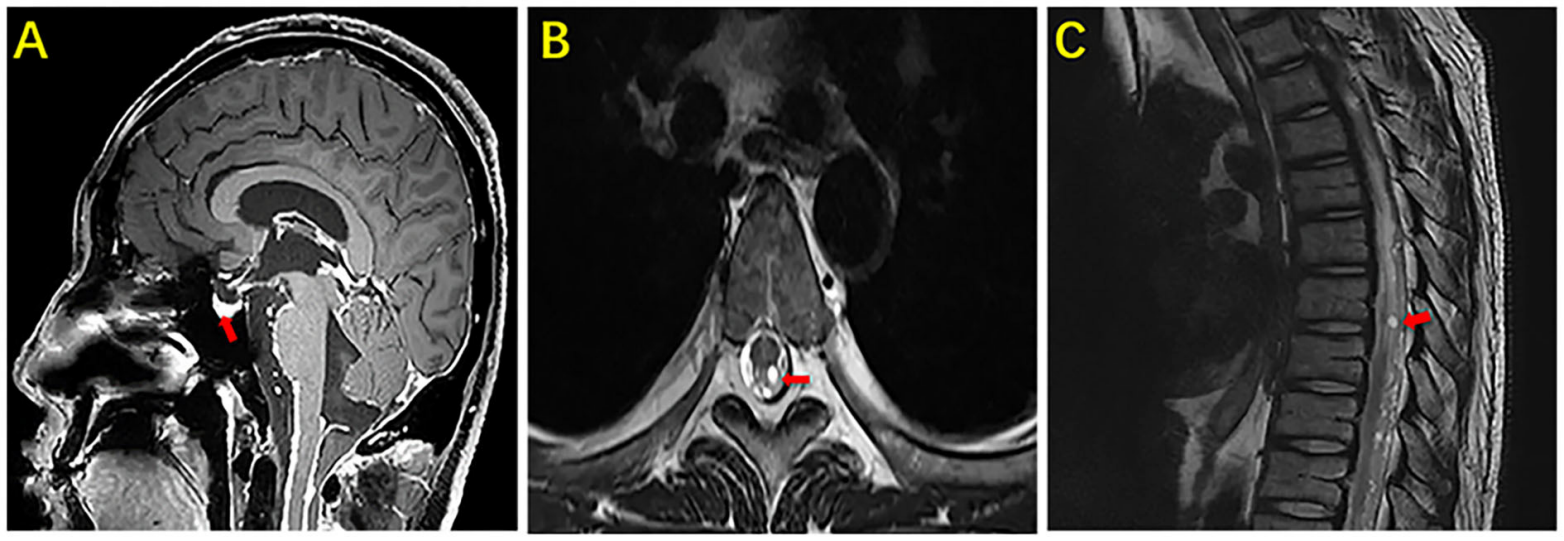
MRI of the brain and thoracic vertebra of our case. The sagittal section enhanced the image of the brain showing marked nodular enhancement under the pia mater (arrow) (A). The axial section and sagittal section of the thoracic vertebra showing marked nodular enhancement in the spinal cord (arrow) (B). The sagittal section of the thoracic vertebra showing marked nodular enhancement in the spinal cord (arrow) (C).
